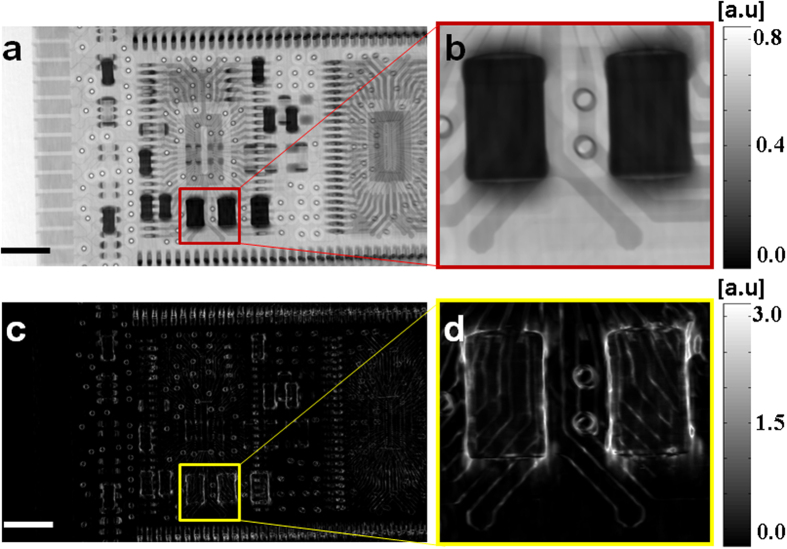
Retrieved transmission and dark-field image of a microchip by using laboratory X-ray microfocus source. (a) Transmission and (c) dark-field image, (b,d) are the magnified parts from image (a,c). The scale bar is equal to 2 mm.
